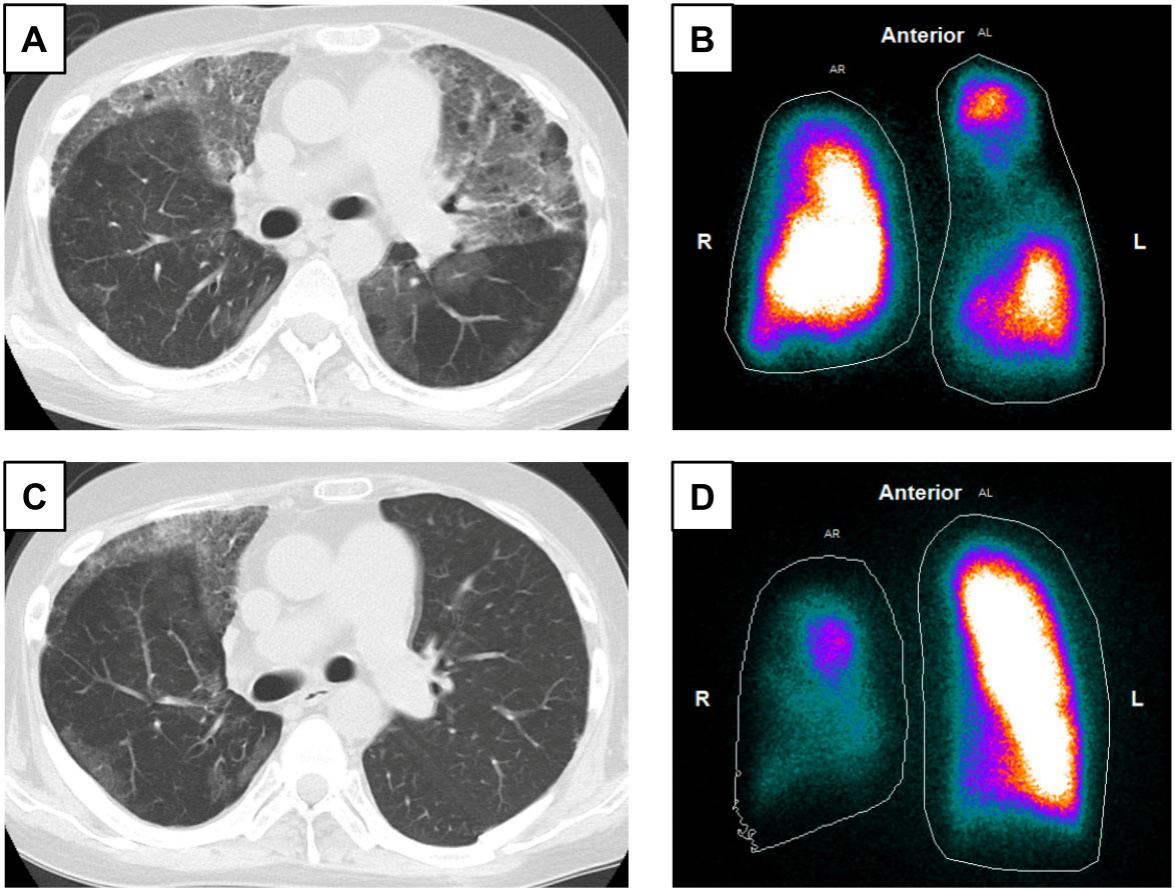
Chest images 9 years after bilateral living donor lobar lung transplantation with sparing bilateral native upper lobes for idiopathic pulmonary arterial hypertension (A, B). Chest computed tomography (CT) showing organizing pneumonia in the spared native upper lobes and bronchiolitis obliterans in the transplanted lower lobes (A). Perfusion scan showing reduction of perfusion in both upper lung fields: 58% of the right and 42% of the left lungs (B). Chest images 9 months after left lung retransplantation from a deceased donor (C, D). Chest CT showing clear left lung field (C). Perfusion scan showing deviation of perfusion to the left transplanted lung: 14% of the right and 86% of the left lungs (D).
